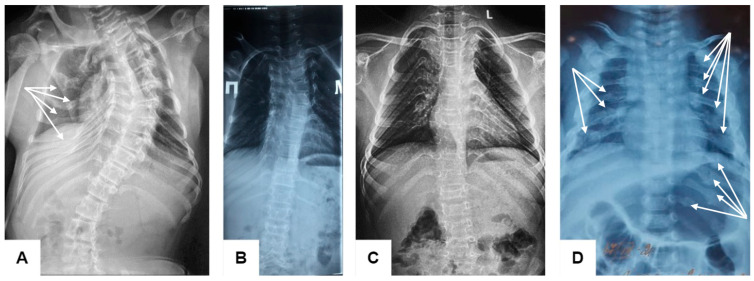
Radiographs of the thoracolumbar spine and chest in anterior views of patients with osteogenesis imperfecta Type XI of different ages ((A)—F1-P1, (B)—F2-P2, (C)—F2-P2, (D)—F4-P4): the chest is narrowed, especially in the upper segment; multiple rib fractures (white arrows); scoliosis of varying severity with a tendency to increase with age.
